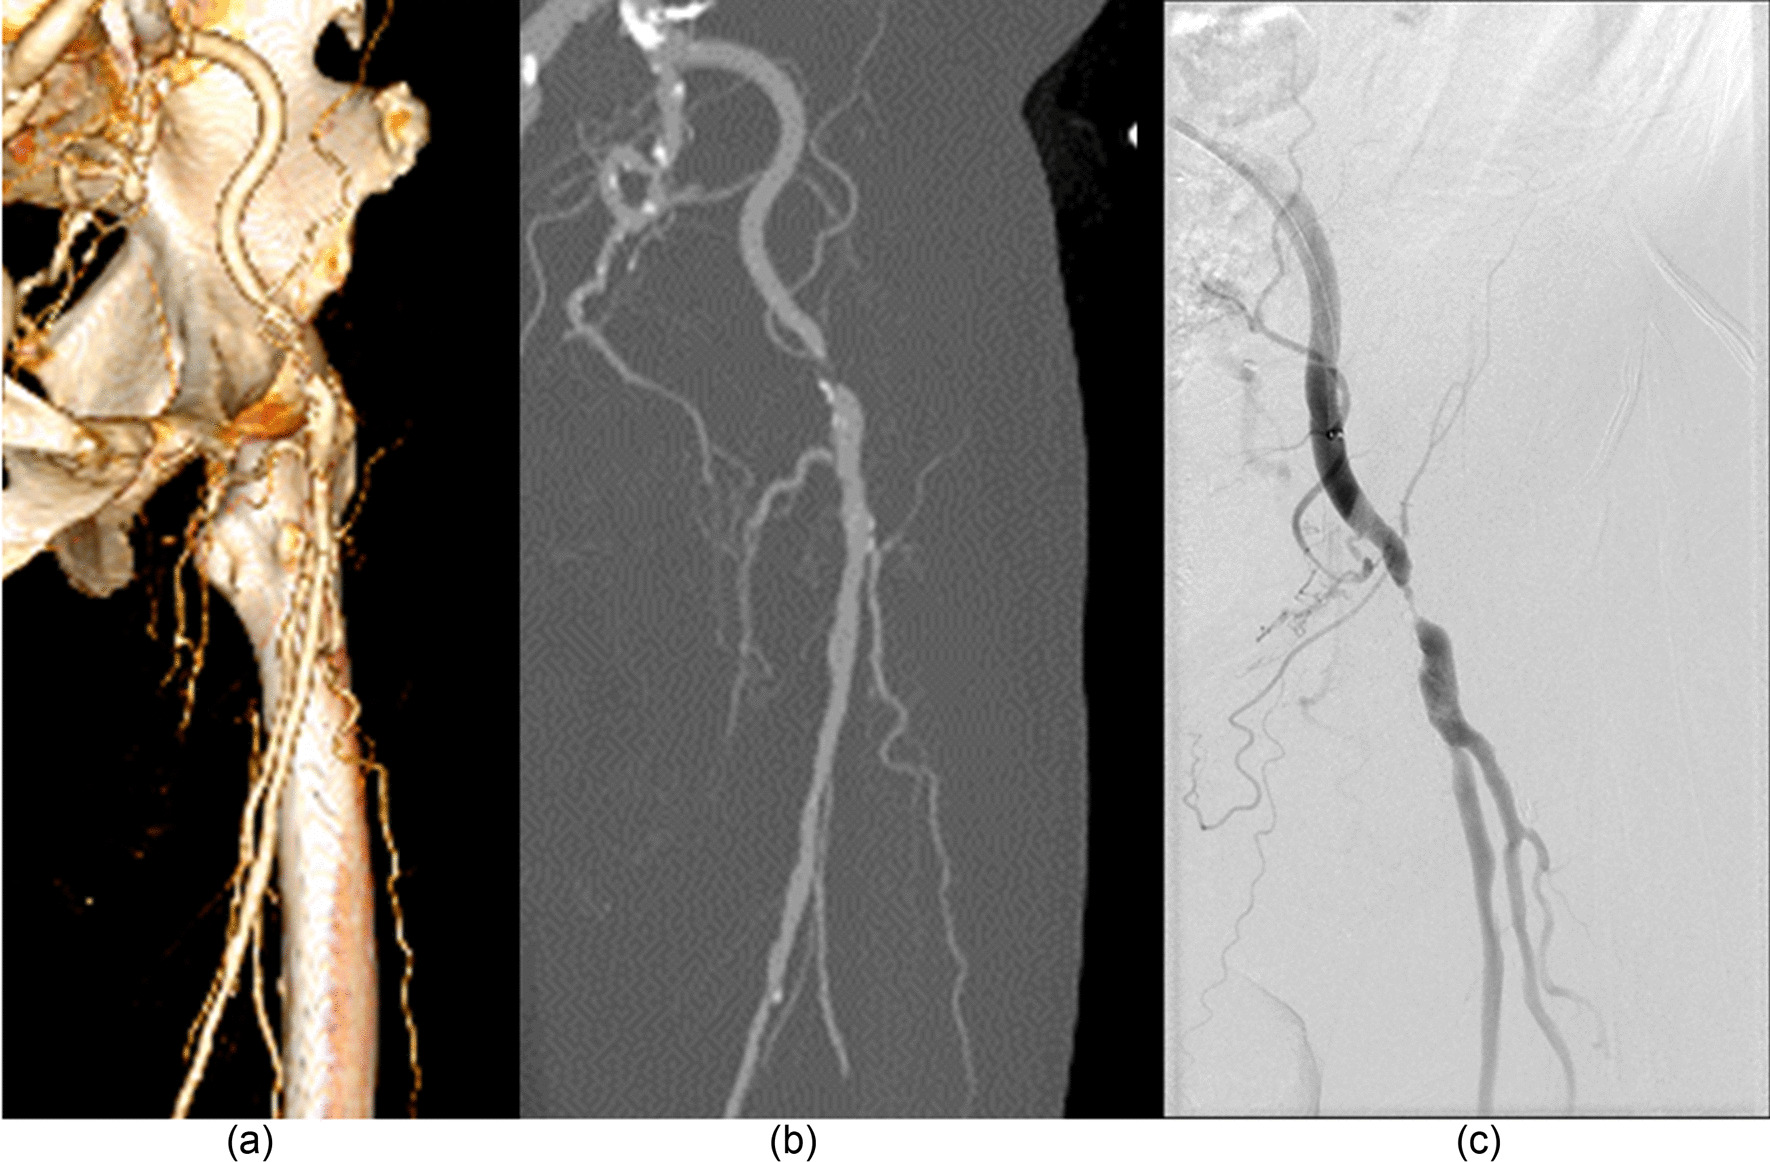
A 77-year-old male with intermittent claudication of both lower limbs for 3 months. There was significant stenosis in the left external iliac artery. Panel a shows the volume rendering CTA image, panel b shows the maximum intensity projection CTA image, and panel c shows the DSA image
